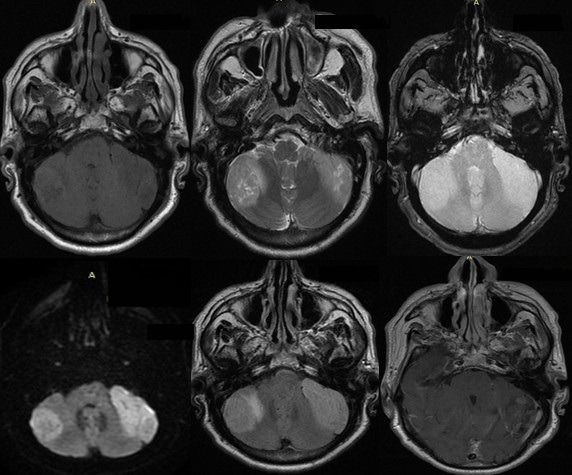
Cerebral MRI: two cerebellar lesions: iso signal weighted T1, hyper signal weighted T2, seat of small area of necrosis, heterogeneously enhanced, with hyper signal diffusion without decrease of the ADC and surrounded by oedema
